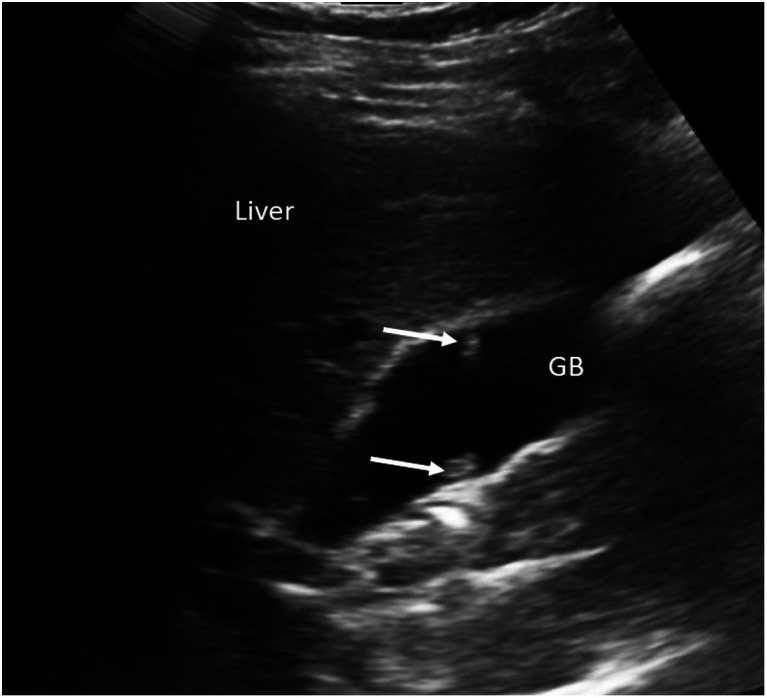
Longitudinal abdominal US of the gallbladder (case number 14) with multiple echogenic polyps with various diameters *(arrows)* in the gallbladder. GB, gallbladder; US, ultrasonography.
